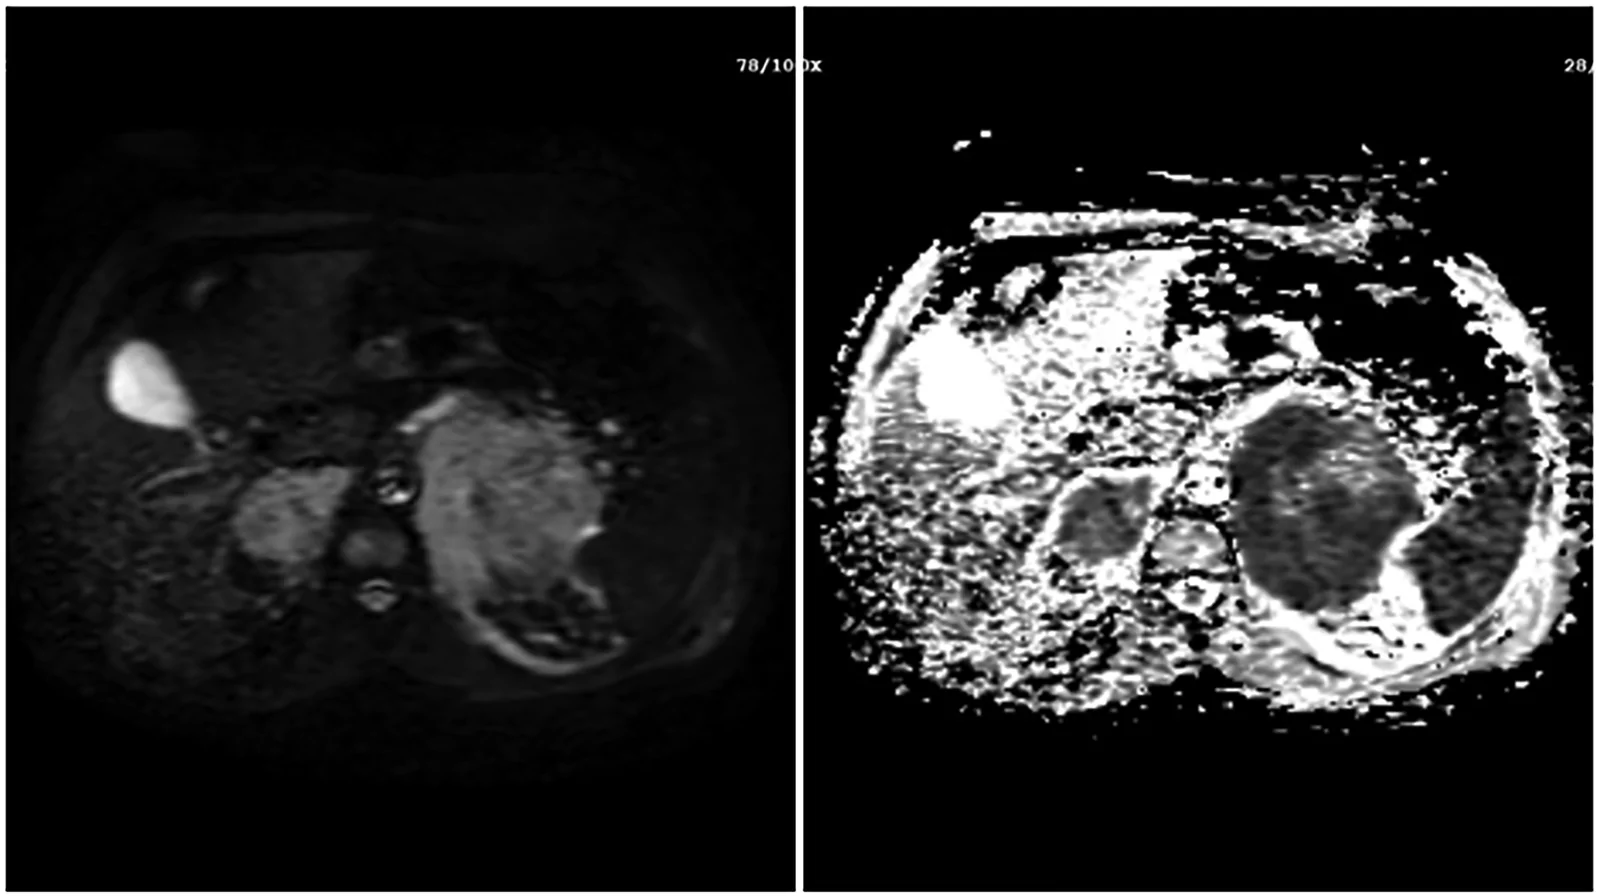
DWI/ADC images showing bilateral adrenal masses with marked diffusion restriction.
